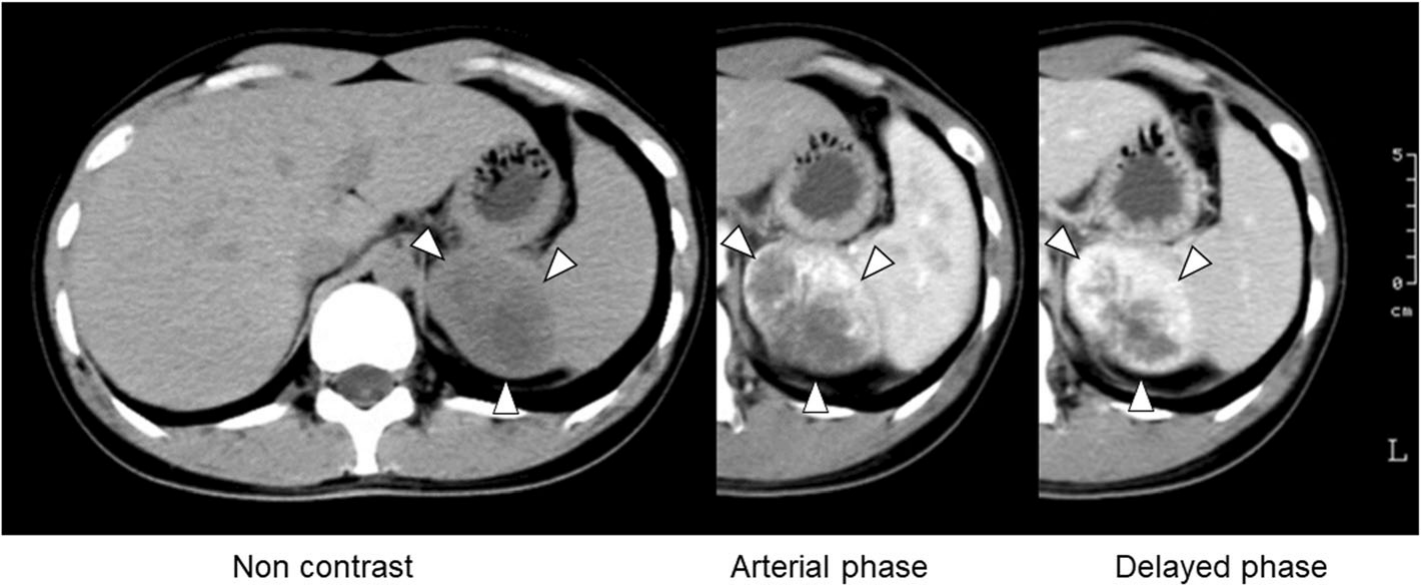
Contrast-enhanced computerized tomography (CT) image. Enhanced computed tomography confirmed the presence of a solid mass measuring about 6 cm with well-defined and regular borders in the retroperitoneum. Peak contrast enhancement in a part of the tumor was seen in the delayed phase
